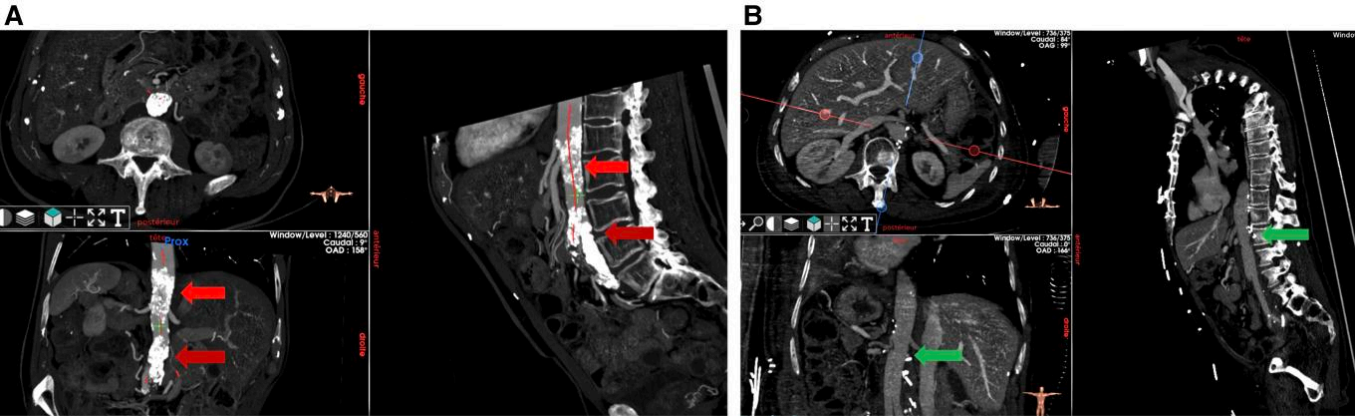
(*A*) Preoperative multiplanar reconstruction scan showing a coral reef of the visceral aorta associated with infrarenal aortic occlusion. The red arrows show the coral reef at the level of the coeliac aorta and at the level of the infrarenal aorta. (*B*) Postoperative CTA of the same patient showing patency of the visceral aorta, renal and digestive arteries after endarterectomy, and aorto-bifemoral bypass. Green arrows show a patent coeliac and infrarenal aorta without residual calcified lesions.
